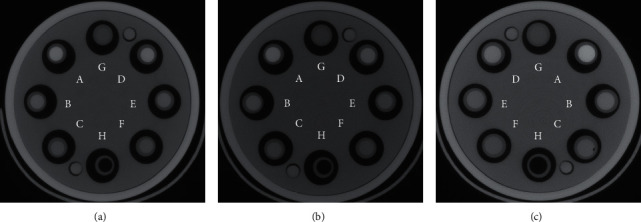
Micro-computed tomography imaging of rhenium and iodine. Representative axial images acquired at 120 kVp by *µ*CT with copper filters with the following thicknesses: (a) 0.6, (b) 1.2, and (c) 1.6 mm. The exposures correspond to X03, X04, and X05, respectively (see Table 1). The images depict the following XCA: Re-XCA ((A) 200 mM, (B) 100 mM, and (C) 50 mM), I-XCA ((D) 200 mM, (E) 100 mM, and (F) 50 mM), and controls ((G) water and (H) air). Attenuation: in (a), (A) 917 HU, (B) 460 HU, (C) 210 HU, (D) 803 HU, (E) 393 HU, (F) 176 HU, (G) 0, and (H) −998 HU. In (b), (A) 942 HU, (B) 473 HU, (C) 218 HU, (D) 661 HU, (E) 321 HU, (F) 138 HU, (G) 1, and (H) -996 HU. In (c), (A) 971 HU, (B) 486 HU, (C) 227 HU, (D) 610 HU, (E) 305 HU, (F) 137 HU, (G) 0, and (H) −998 HU.
